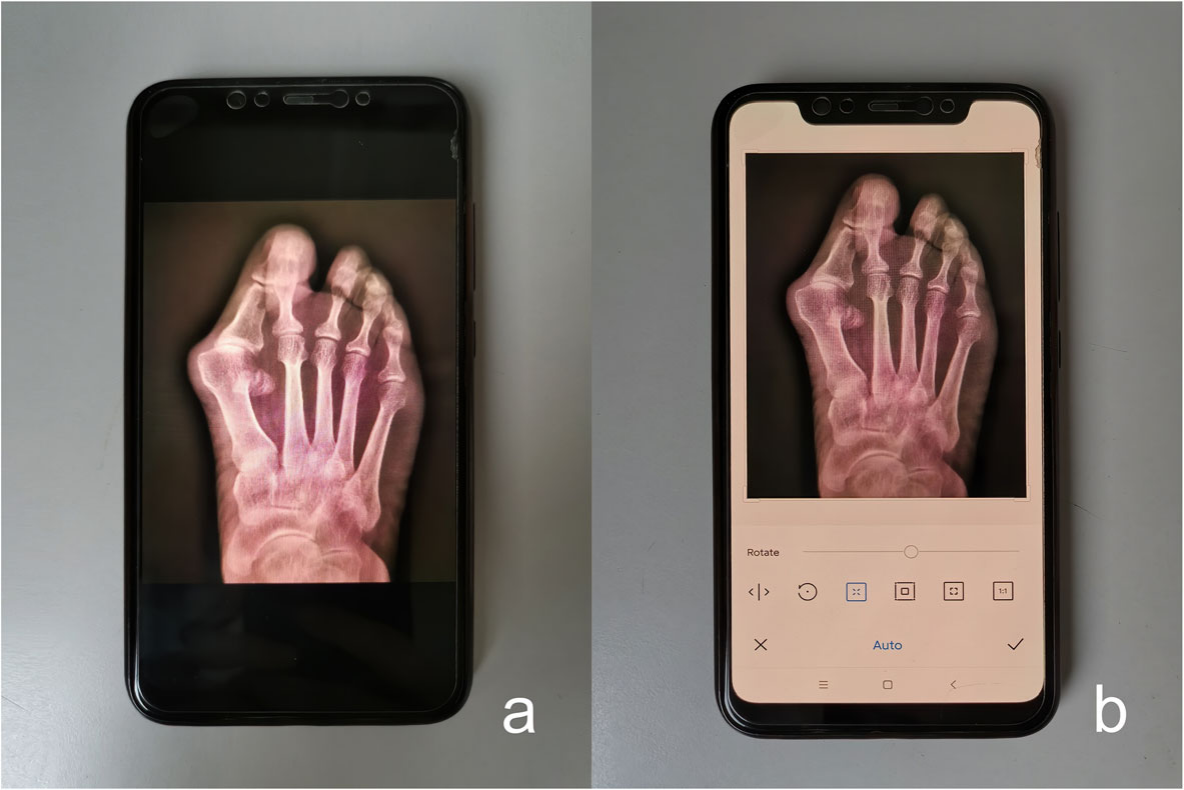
a. The targeted photo was shown on the smartphone. b. The intrinsic photo-editing system was used to rotate the photos of radiographs
